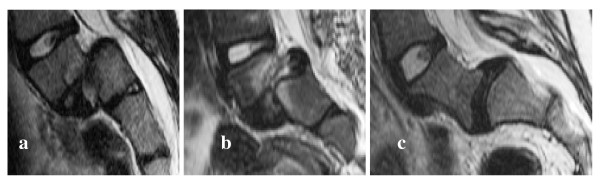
T2-weighted MR images of intervertebral discs L5/S1 of patients suffering from spondylolisthesis. The disc is cut in two parts (a and b) or the high intensity zone representing the nucleus does not exist (c). The patients were all females, 15 (a), 13 (b) and 16 (c) years old.
